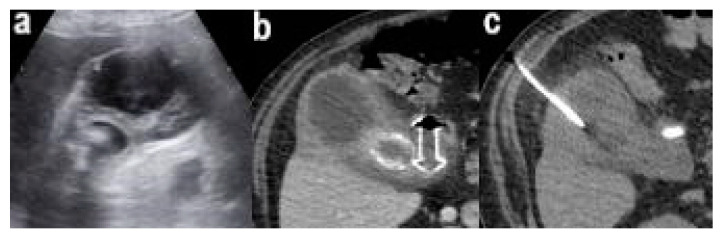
Abdominal US showed an ACC with a 50 mm abscess of the wall and the known big gallstone 37 × 30 mm (a), CT scan show Hot-Axios positioned between the gallbladder and duodenum (b), CT scan-guided PC-GBD (c).
